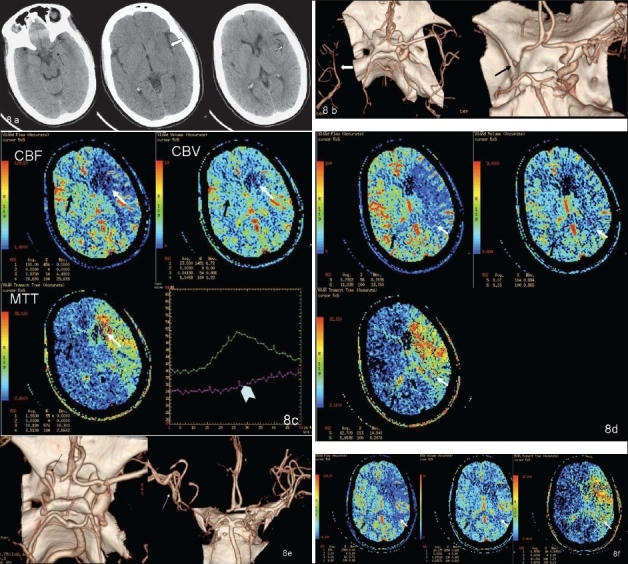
Initial NCCT (A) (at 1.5 h from stroke onset) showing hyperdense left MCA (black arrow), loss of insular ribbon (white open arrow), and obscuration with subtle hypodensity of the left lentiform nucleus (curved arrow). CTA volume-rendered images (B) show complete occlusion of the left intracranial ICA and M1 segment (black arrow on the right); note poor filling of the distal MCA branches, possibly through leptomeningeal collaterals (white arrow in the left image). Color-coded perfusion maps (C) for CBF, CBV, and MTT- ROI (white arrow) placed in the ischemic core—umbra—with mirror ROI (black arrow) in the contralateral hemisphere; relative values for CBF, CBV, and MTT are shown as a percentage of normal hemispheres (left hand bottom corner of each map). Time–attenuation curve showing lack of contrast arrival peak in the ROI on the affected side (arrow head). Color-coded perfusion maps (D) for CBF, CBV, and MTT- ROI (white arrows) placed in the ischemic penumbra, with mirror ROI (black arrows) in the contralateral hemisphere. The values (left hand bottom corner of each map) of rCBF and rCBV are greater, and rMTT lesser, than those in the umbra (see C). Post-thrombolysis CTA volume-rendered image (E) showing partial recanalization of the left cavernous ICA (black arrow) with occluded supraclinoid ICA and M1 segment and better filling of distal MCA branches (white arrow) as compared to the pre-thrombolysis CTA (see B). Color-coded perfusion maps (F) for CBF, CBV, and MTT- ROI (white arrow) placed in the ischemic penumbra, with mirror ROI (black arrow) in the contralateral hemisphere. rCBF and rCBV values are higher than the pre-thrombolysis values (left hand bottom corner of each map). However, there is no significant change in the size of the penumbra as compared to the prethrombolysis perfusion CT
